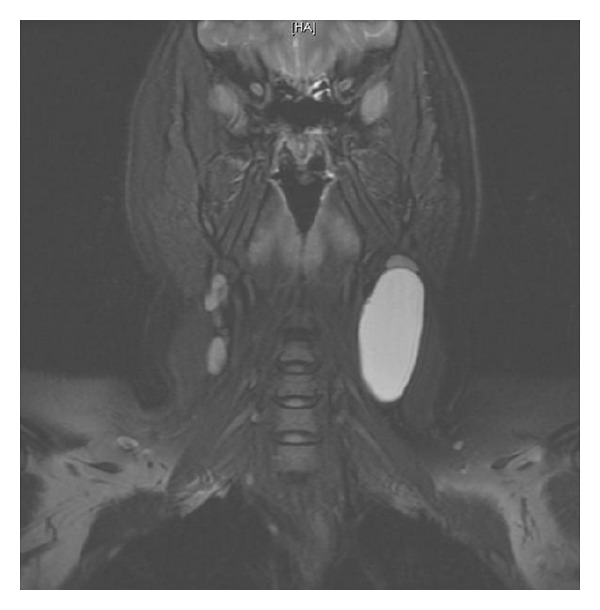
MRI-coronal view showing the cystic lesion with high T2 signal.
